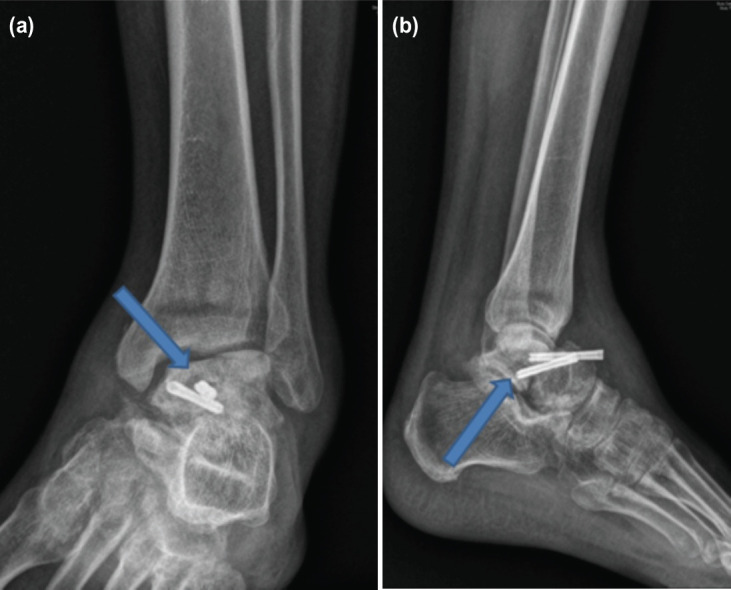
(a, b) Pre-operative AP and lateral radiographs of Patient 4.
